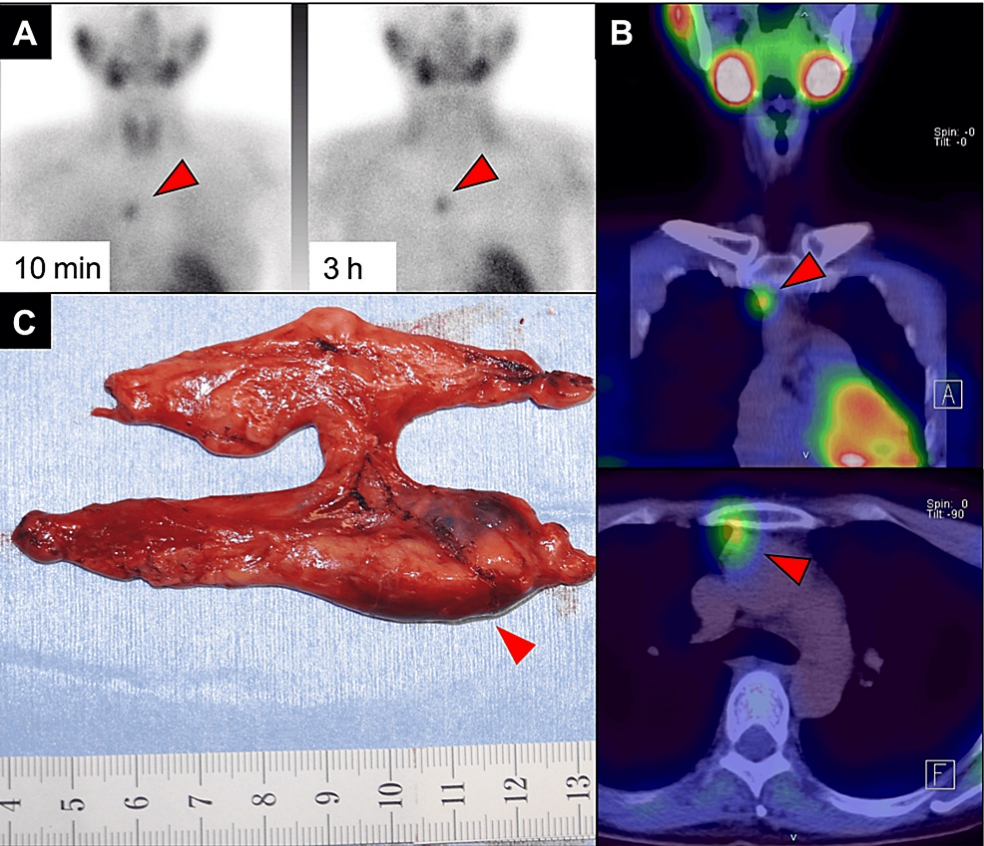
Diagnostic and surgical visualization of parathyroid adenoma with 99mTc-MIBI scintigraphy, SPECT/CT, and gross pathology. Slices of technetium-99m-labeled methoxy isobutyl isonitrile (99mTc-MIBI) scintigraphy. A Symbia T6 (Erlangen, Germany: Siemens Healthineers) single-photon emission computed tomography/computed tomography (SPECT/CT) system was used. (A) A whole-body scan of anteroposterior (AP) view is shown. An early phase of 10 min after injection (left) and delayed phase of 3 h after injection (right) were shown. The focus of intense activity in the right anterior mediastinum (arrowhead) corresponds to the parathyroid adenoma of the patient described in the text. (B) Delayed phase SPECT/CT fusion image. The spot in the mediastinum (arrowhead) corresponds to the parathyroid adenoma of the patient described in the text. (C) Gross image of the extracted thymus containing the nodule of parathyroid adenoma (arrowhead). The thymus and nodule were excised en bloc.
